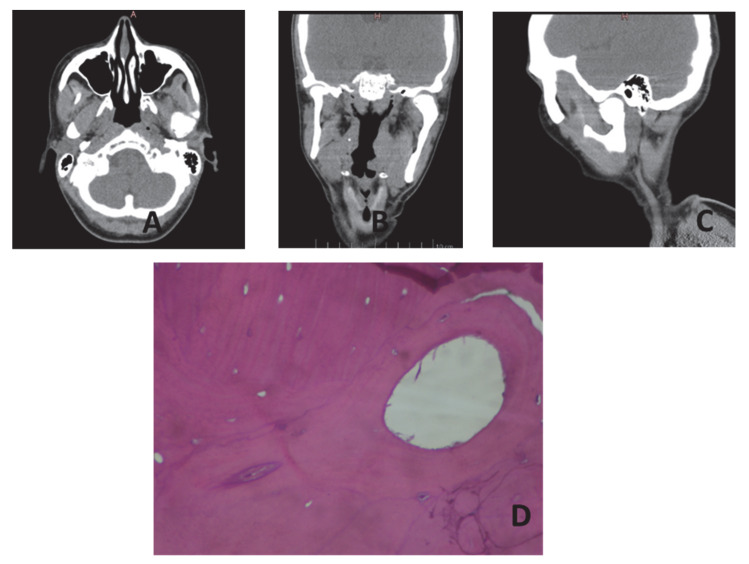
(A) Transverse section of CT imaging. There is an obvious enlargement of the left mandibular condyle; (B) Coronal view showing the affected left side in comparison with the healthy right side; (C) Oblique section of CT imaging. The enlarged left condyle is depicted; (D) Condylar osteoma consisting of osteocytes and haversian canals H & E X400.
